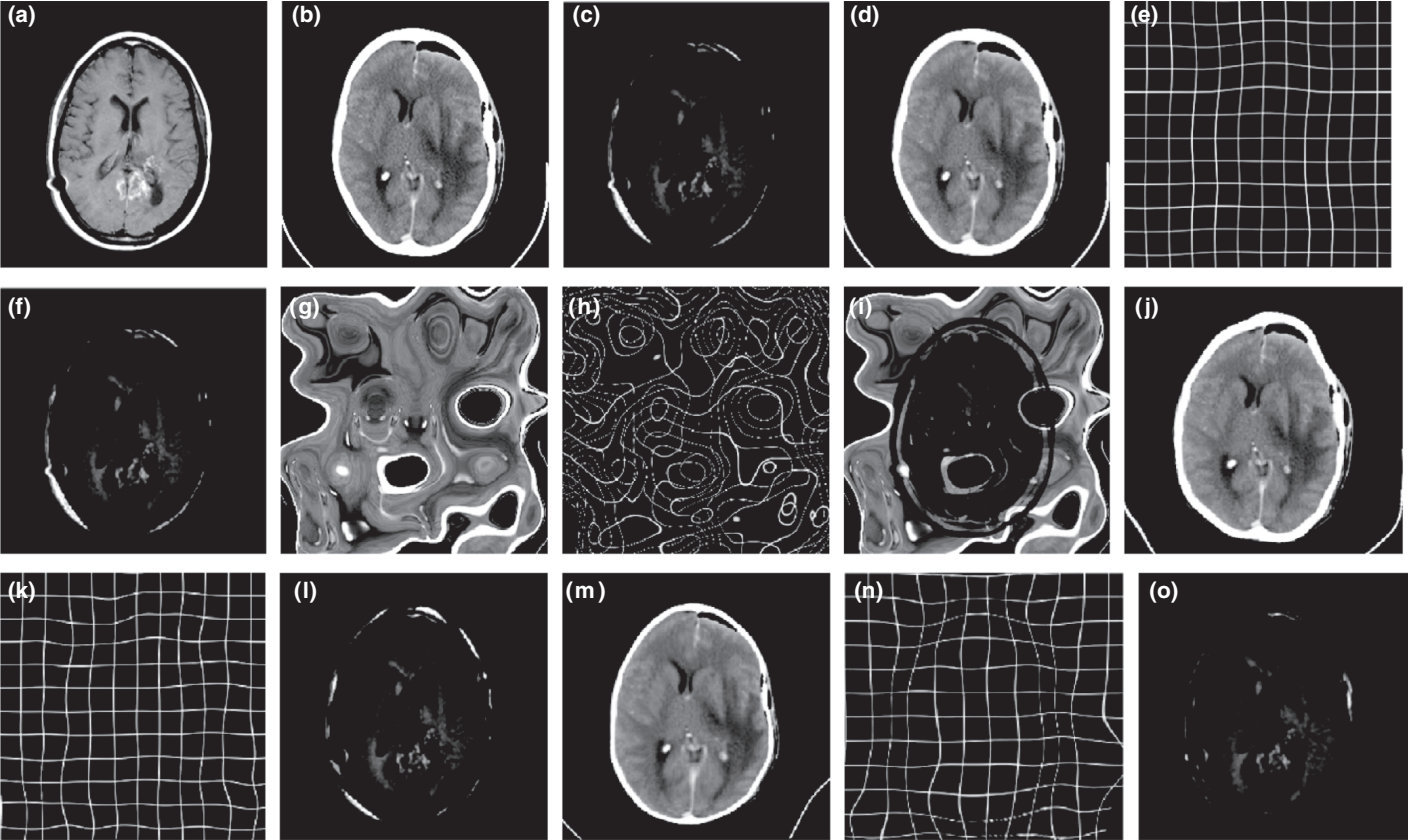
Brain image multimodality registration result. (a) MRI reference image; (b) CT floating image; (c) registration error (a) and (b); (d) gradient difference (GD) deformed image; (e) GD deformation field; (f) registration error (a) and (d); (g) normalized mutual information (NMI) deformed image; (h) NMI deformation field; (i) registration error (a) and (g); (j) local structure tensor‐normalized mutual information (LST‐NMI) deformed image; (k) LST‐NMI deformation field; (l) registration error (a) and (j); (m) adaptive local structure tensor‐normalized mutual information (ALST‐NMI) deformed image; (n) ALST‐NMI deformation field; (o) registration error (a) and (m).
